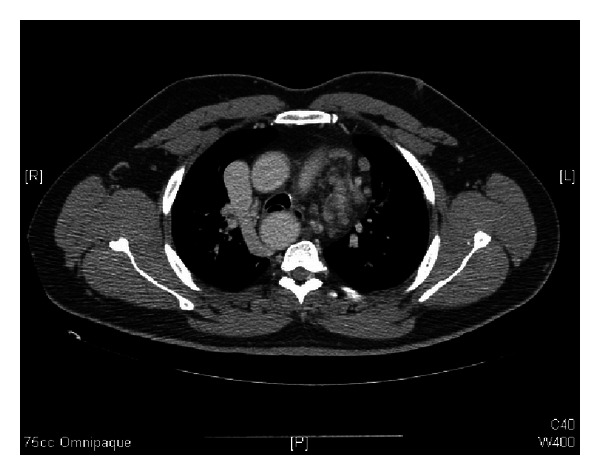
Axial CT scan at T5 revealing the presence of an incidental right-sided thoracic aorta.
